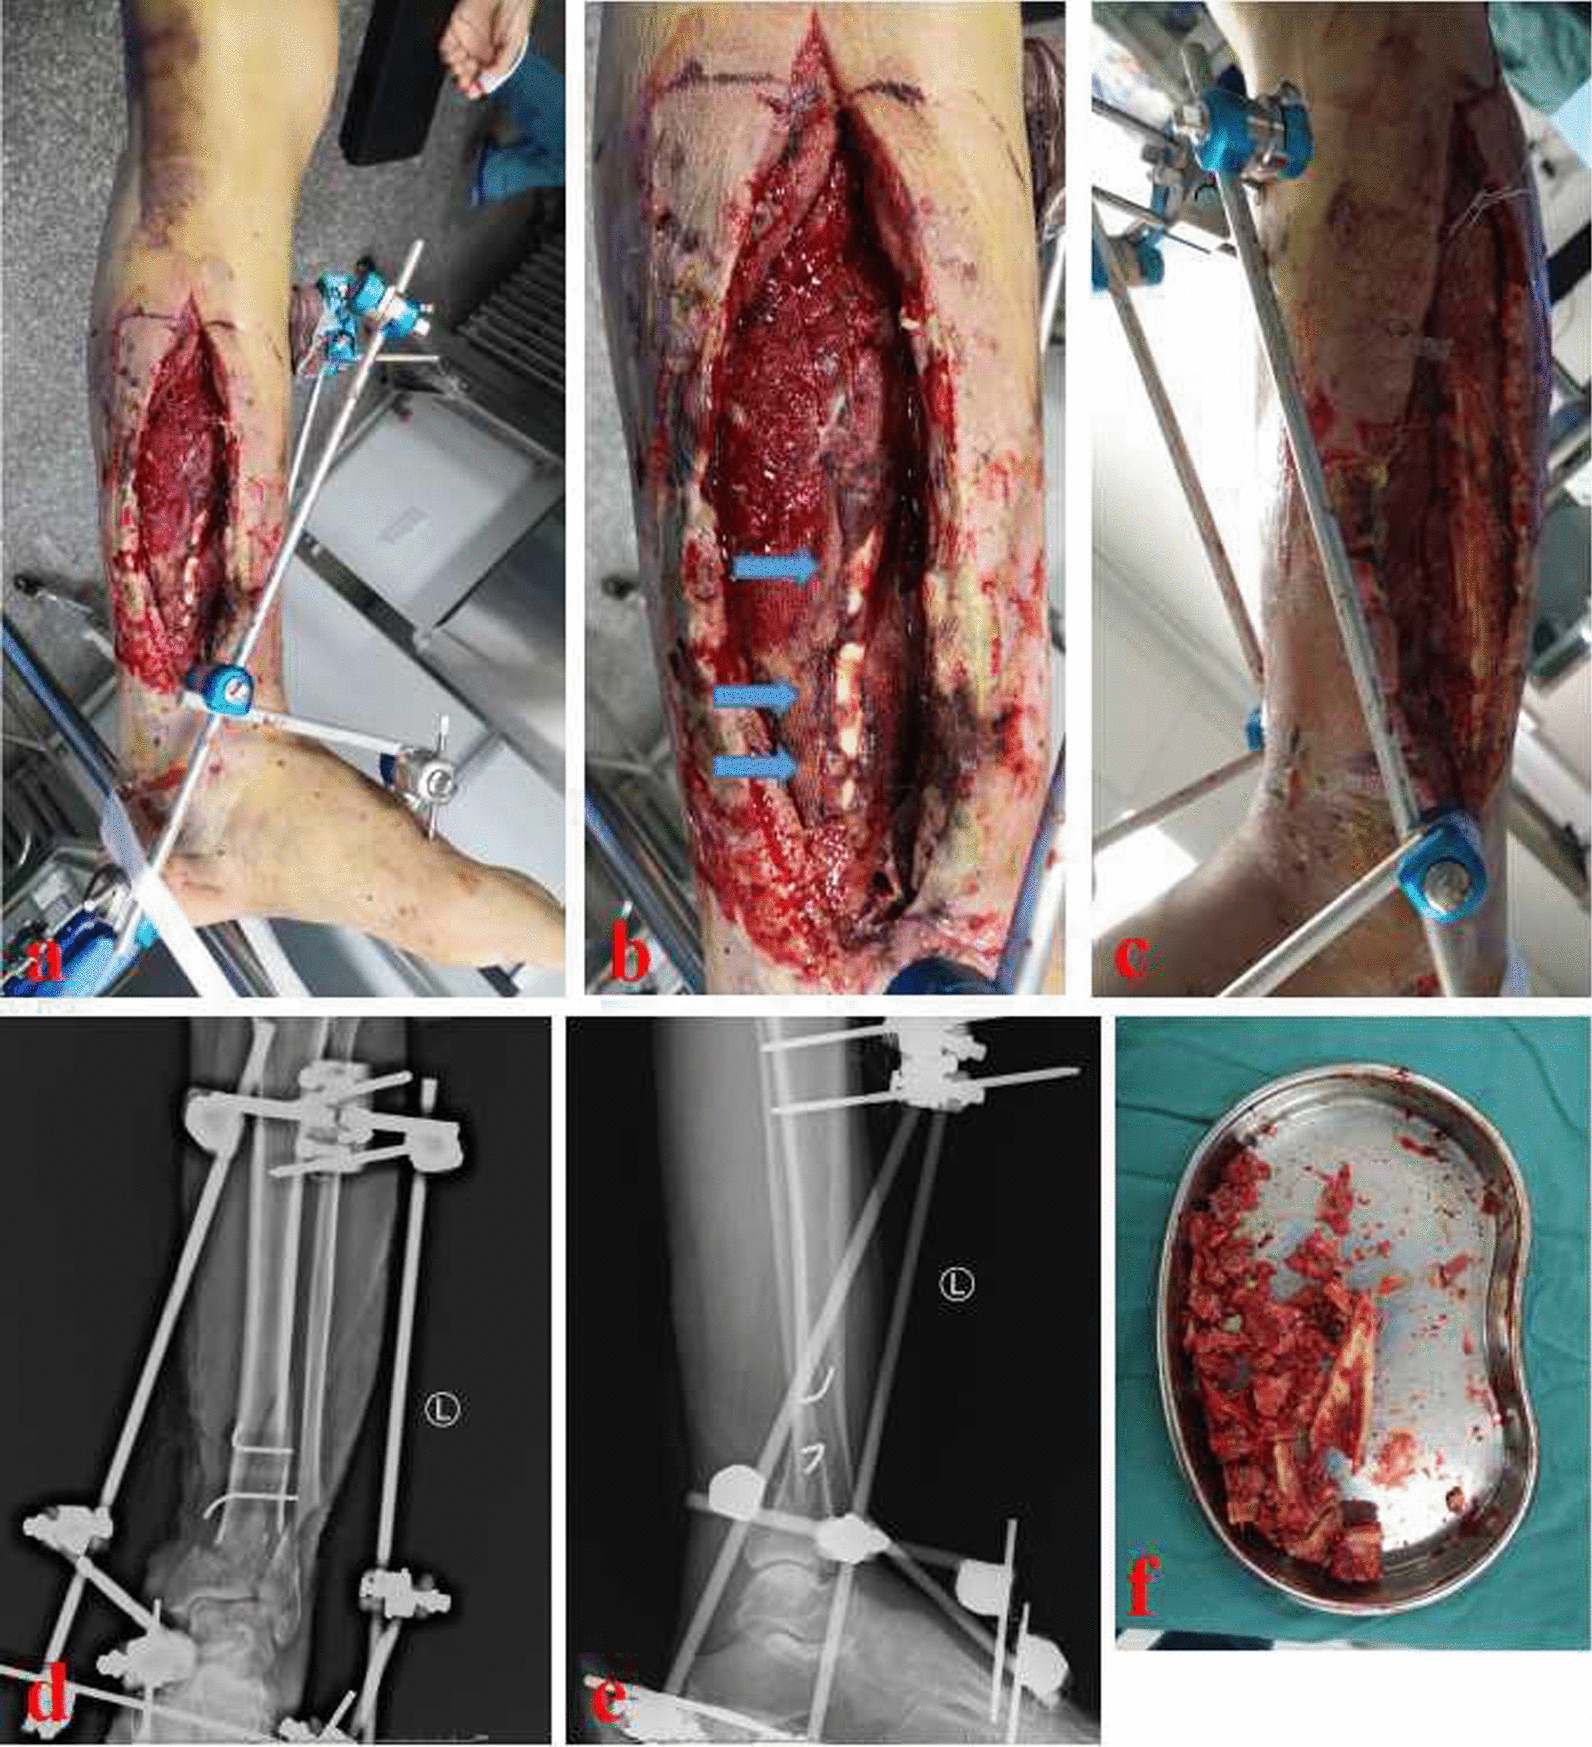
A 51-year-old male suffered from osteofascial compartment syndrome and distal tibial osteomyelitis. a–c When the patient came to our hospital, the initial appearance of the injured leg was recorded. Blue arrows indicate infected bones at the distal tibia; d and e Initial X-ray films of the distal tibia; f The plate shows sequestrum and infected bone tissues removed during the first debridement in our hospital
